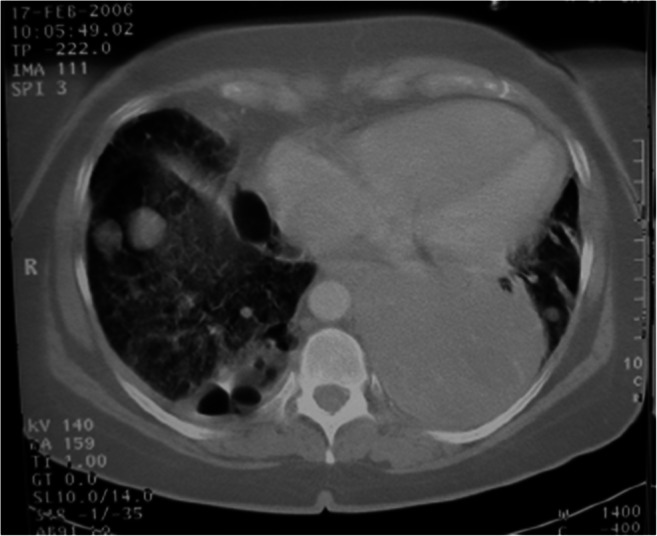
CT scan showing the large tumor in the left lung of patient 1
